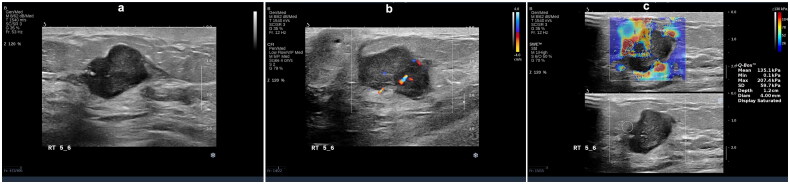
36-year-old female with left breast invasive ductal carcinoma grade III (score of 8), tumor size 2.2 × 1.6 × 1.3 cm, HER2+ subtype. (a) Ultrasonography features: irregular shape, lobulated margins, no change in posterior echo, multiple punctate calcifications. (b) Adler blood flow grading – grade 2. (c) Emax = 207.4 Kpa.
